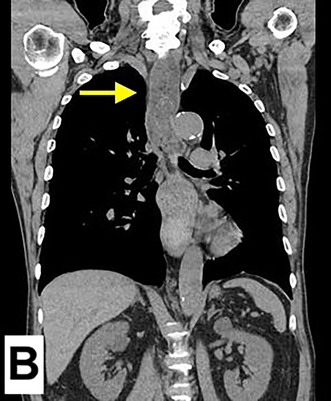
A 77‐year‐old man presented to the emergency department with chest constriction and persistent vomiting after consuming chopped octopus. A non‐contrast CT scan revealed esophageal dilation with a hyperdense mass obstructing the esophagogastric junction (Figure 1A) and food residues extending into the cervical esophagus (Figure 1B), suggesting significant obstruction and increasing the risk of aspiration. To reduce this risk and improve visibility during endoscopy, a nasogastric tube was inserted approximately 30 cm through the nasal passage, and approximately 50 mL of yellowish, muddy food residues were aspirated. Subsequent esophagogastroduodenoscopy identified an octopus lodged in the distal esophagus (Figure 2). Attempts to remove the octopus using forceps or a retrieval basket were unsuccessful due to its slippery texture, which caused it to disintegrate. Consequently, the push technique^1^ was employed, successfully advancing the octopus into the stomach and confirming its passage through the esophagogastric junction. Post‐procedural evaluation confirmed that both the stomach and esophagus were intact.
